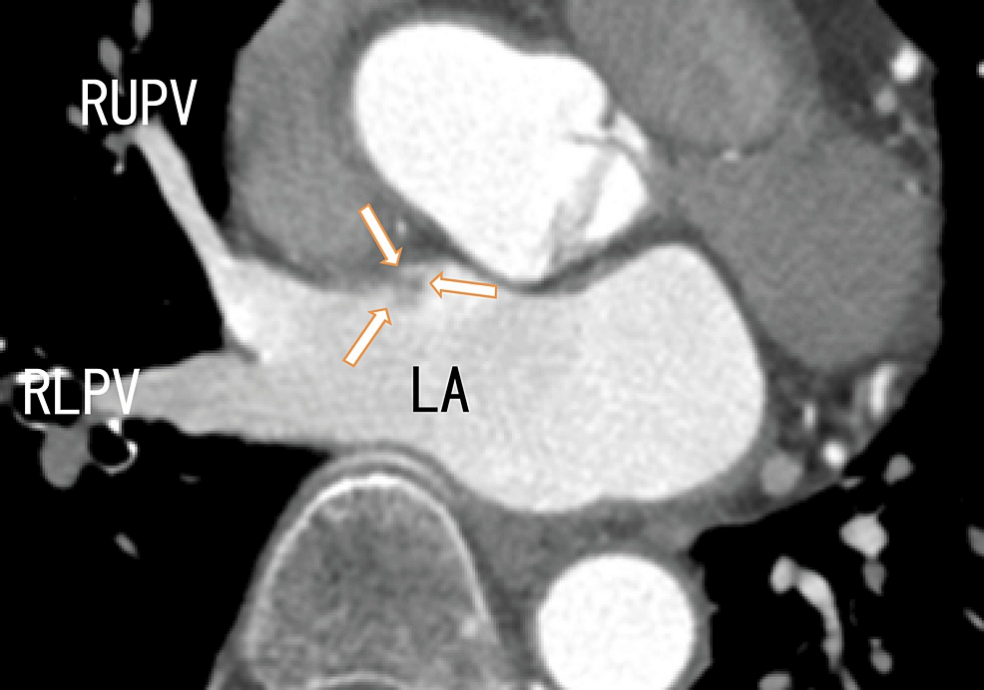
Axial images from an 80-MDCT scan revealing the RUPV, RLPV, and LA There were no images of thrombi in the LA. There was a rather dark area in the RLPV, and there was a dark line in the center of the RUPV, indicating that there were thrombi in both the RUPV and the RLPV. Additionally, there was a strongly dark area in the LA (arrows), suggesting that both extended RLPV thrombi and the anterior wall of the LA were attached. LA, left atrium; RLPV, right lower pulmonary vein; RUPV, right upper pulmonary vein, MDCT, multidetector computed tomography
